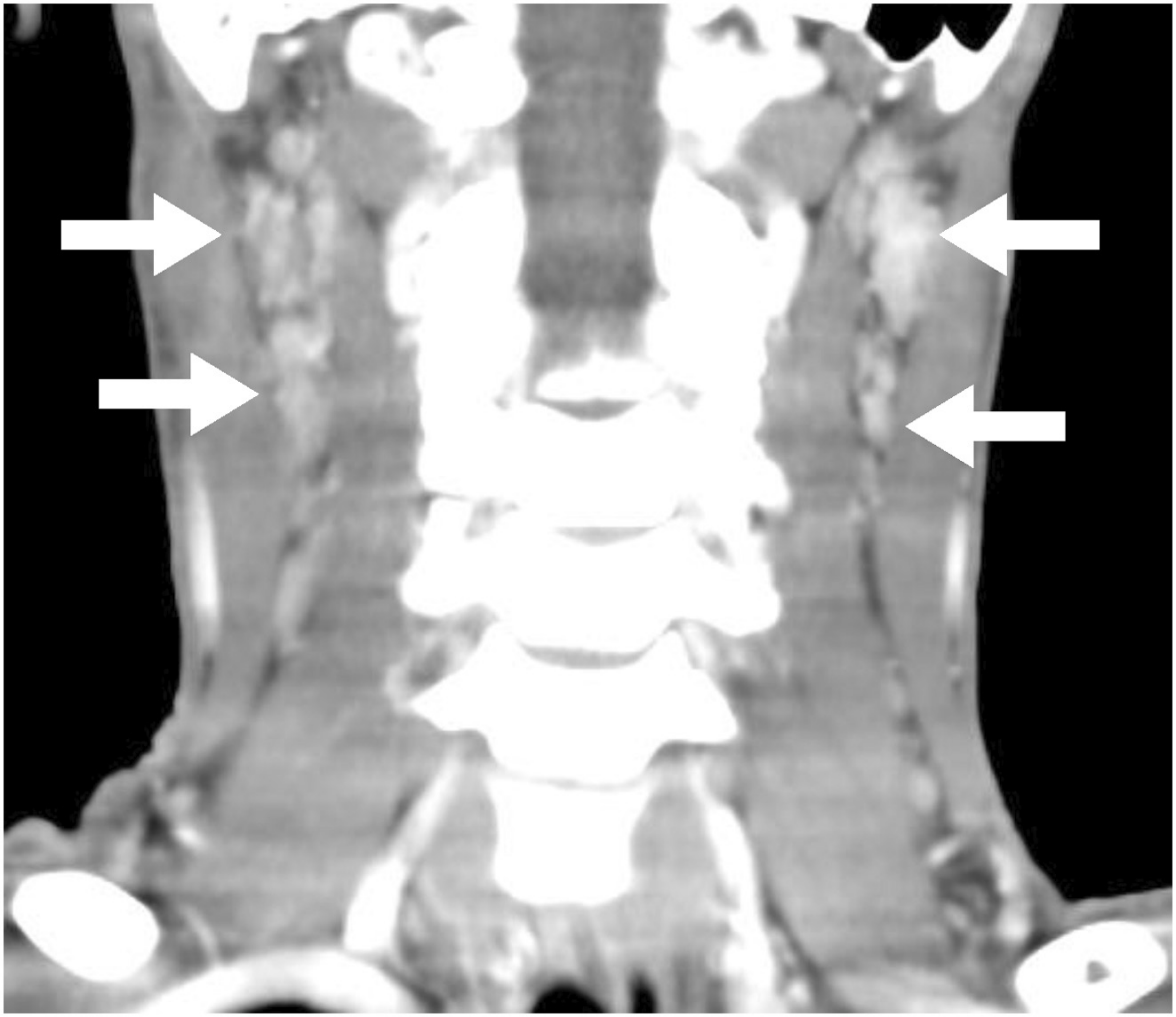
CT image of the bilateral neck lymphadenopathy (arrows)
